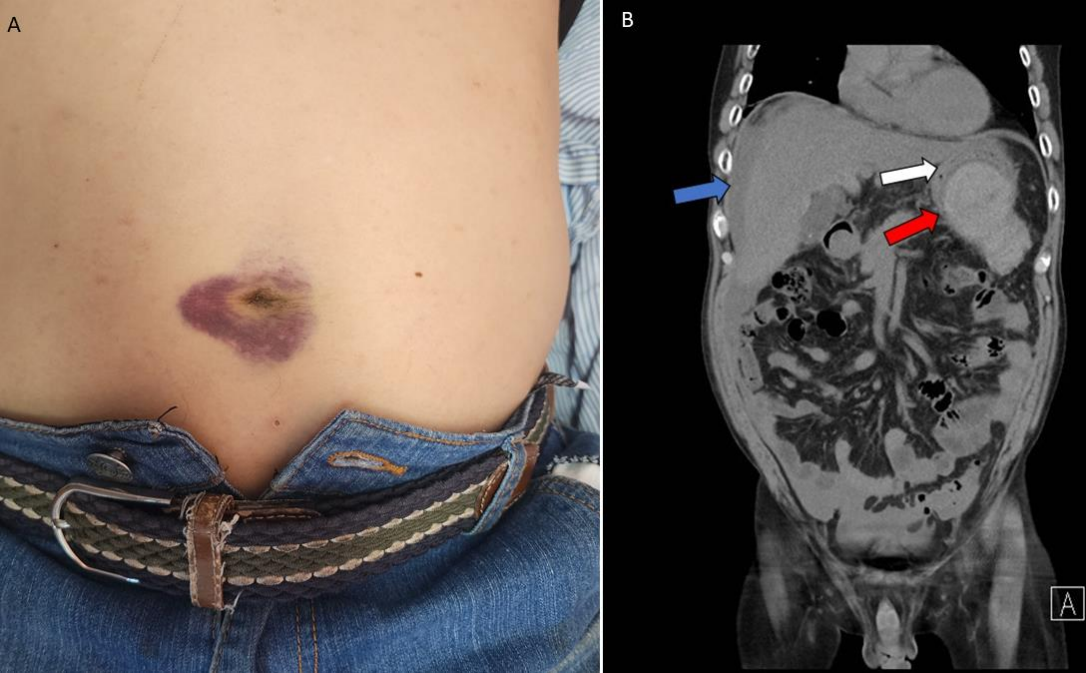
A) periumbilical ecchymosis consistent with Cullen sign; B) contrast-enhanced abdominal CT showing a hemorrhagic pancreatic cyst (red arrow) with associated hemoperitoneum (blue arrow) and compression of the stomach (white arrow)
